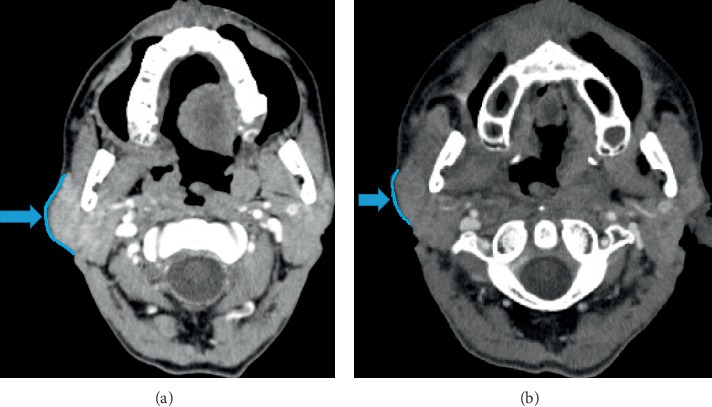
Enhanced-contrast computed tomography (CECT) showing the convexity of the parotid gland prior to chemoradiation therapy (CRT) (a). CECT showing the tendency of the parotid gland to flatten in the post-CRT period (b). The CRT resulted in a migration of the parotids toward the midline (medial) and superior (cranial) directions. This migration can be explained by the fact that the lateral boundaries of the gland suffer more directly by the effects of radiation, considering it is not surrounded by bone structures. Therefore, the gland loses its convexity and tends to acquire a flat or even concave shape.
